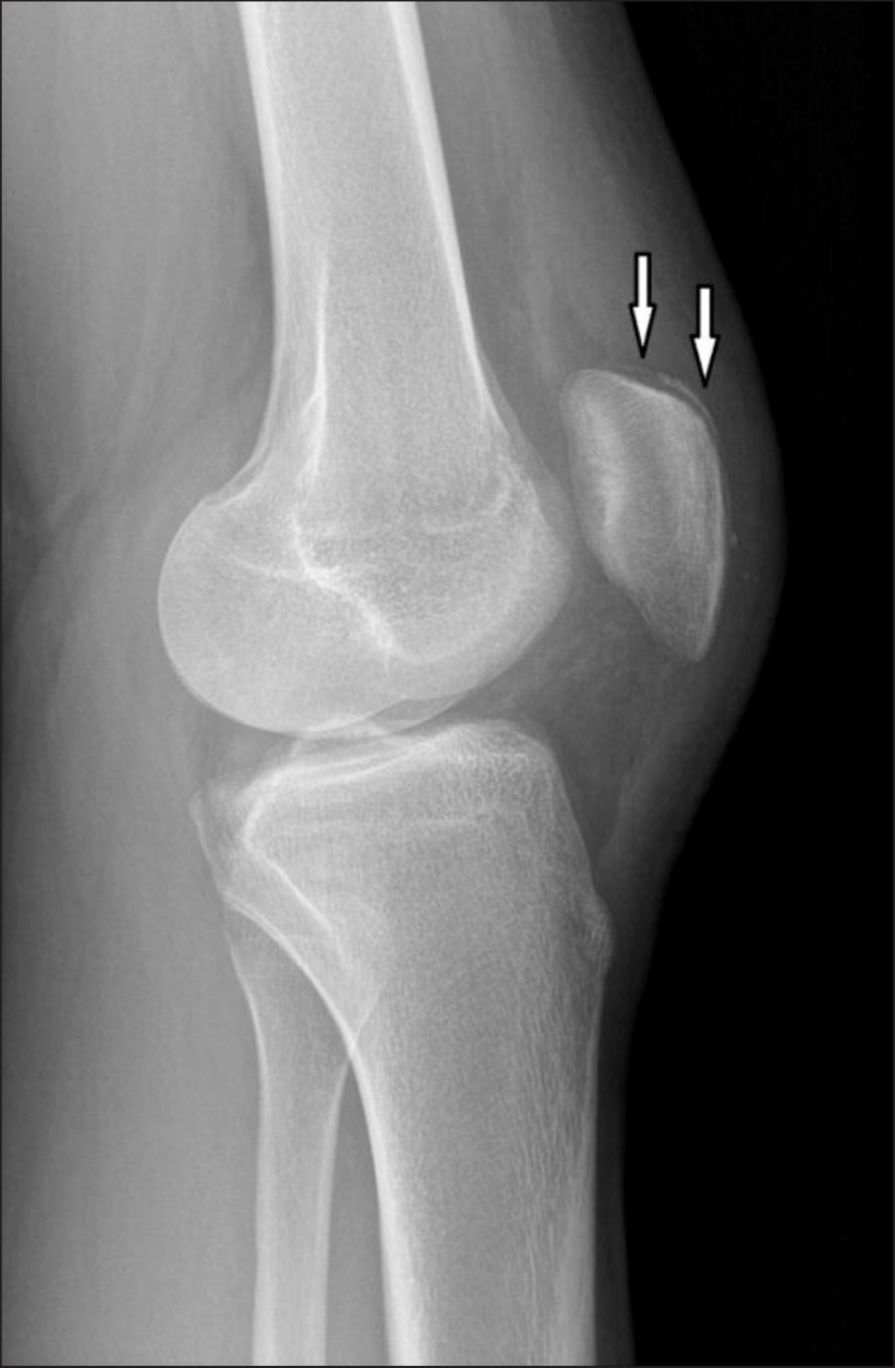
Lateral X-ray of the knee shows a linear bony fragment at the upper pole of the patella.
